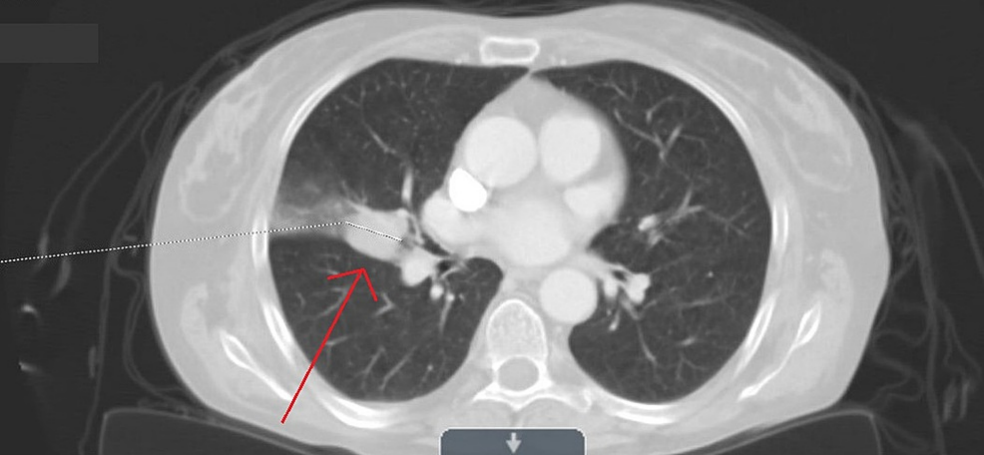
Chest CT showing a round 1.7 cm pulmonary nodule in the right perihilar region with adjunct ground-glass opacity. CT: computed tomography.
